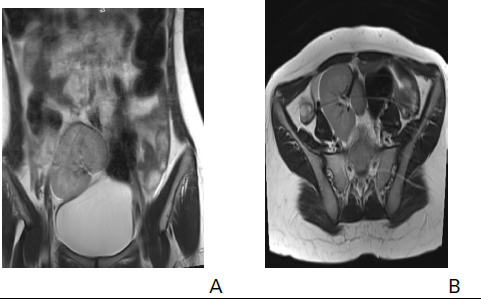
Coronal and axial T2 weighted MRI showing both the kidneys fused together within the pelvic cavity with single renal pelvis giving pancake appearance (A) and (B).
